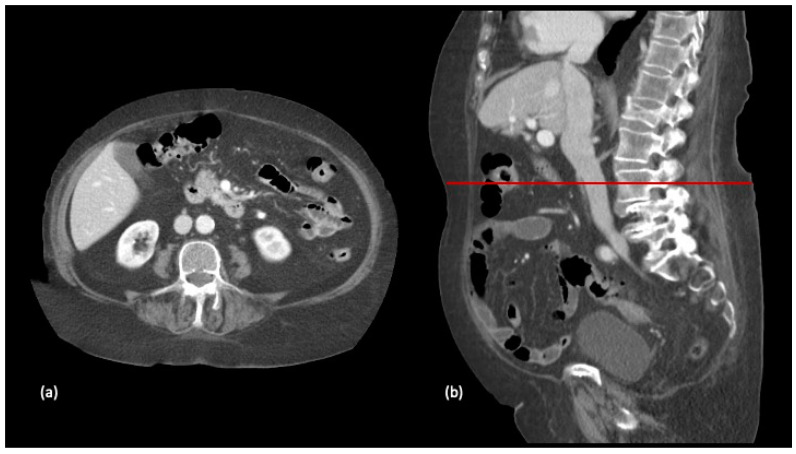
Selection of axial (a) and sagittal (b) slices at the level of the third lumbar vertebra in a preparatory computed tomography study.
